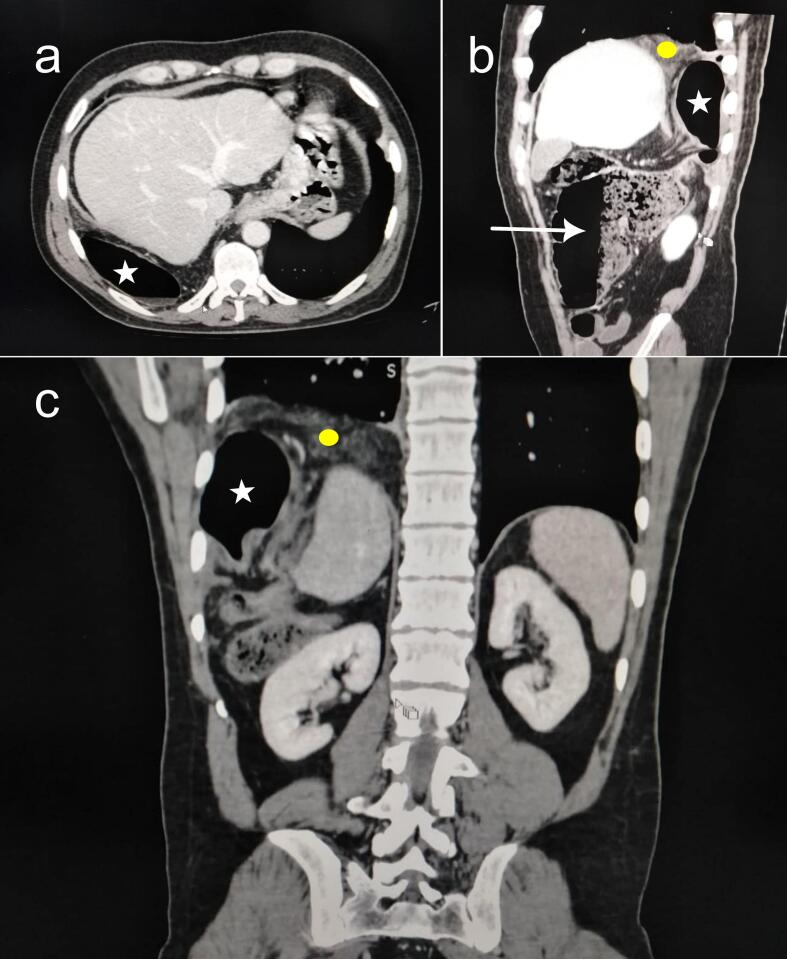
Preoperative CT scan. Portal phase (a) axial view, (b) sagittal view, and coronal view showing the incarcerated right colic flexure (white stars) and omentum (yellow circles). The ascending colon (white arrow) is distended as shown in (b). (For interpretation of the references to colour in this figure legend, the reader is referred to the web version of this article.)
